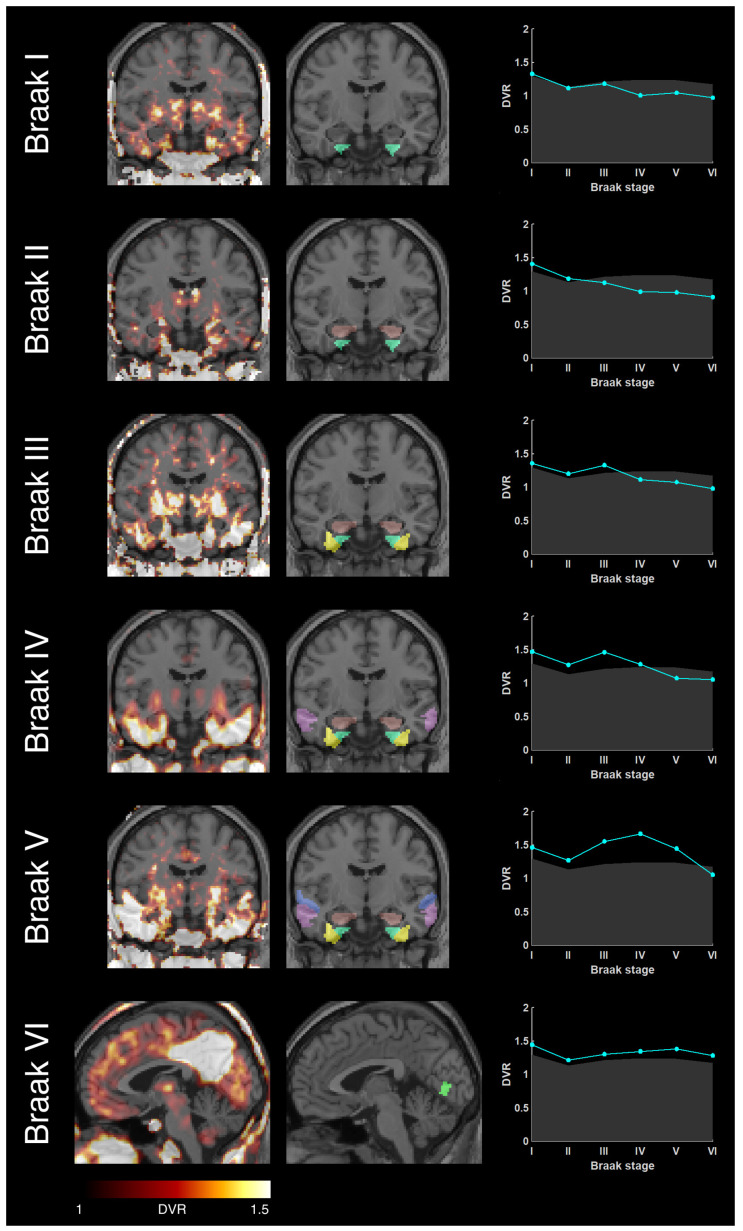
Example AD dementia cases for each Braak stage showing parametric tau PET image fused with a standard MRI (left), MRI including Braak atlas regions (middle) and individual Braak profile (right). The plot shows mean DVR values of each Braak stage (cyan) in relation to the normal DVR (gray) defined by the healthy controls.
